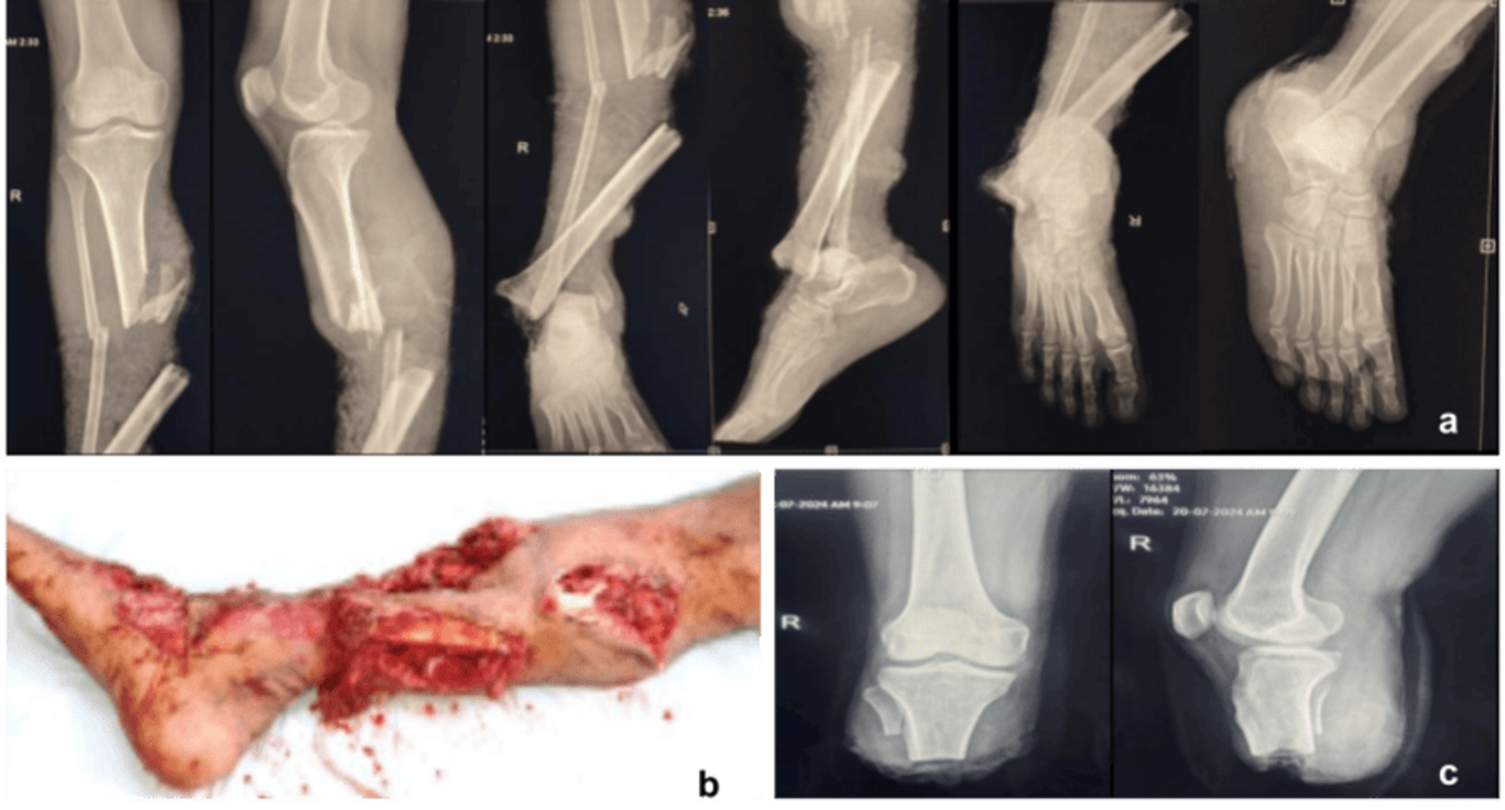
Radiological and clinical findings in a patient undergoing below-knee amputation. A 30-year-old male patient managed with wound debridement and dressing followed by right-sided below-knee amputation. (a) Pre-operative anteroposterior and lateral radiographs demonstrating the extent of bony injury. (b) Pre-operative clinical photograph showing severe soft tissue damage. (c) Post-operative radiograph confirming completion of below-knee amputation with satisfactory stump formation.
